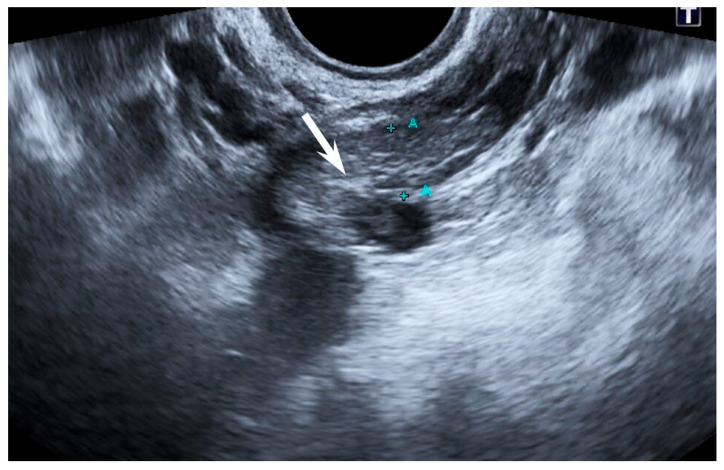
Duplex ultrasound scanning. Blood clot in the lumen of a parametrial vein (arrow).
